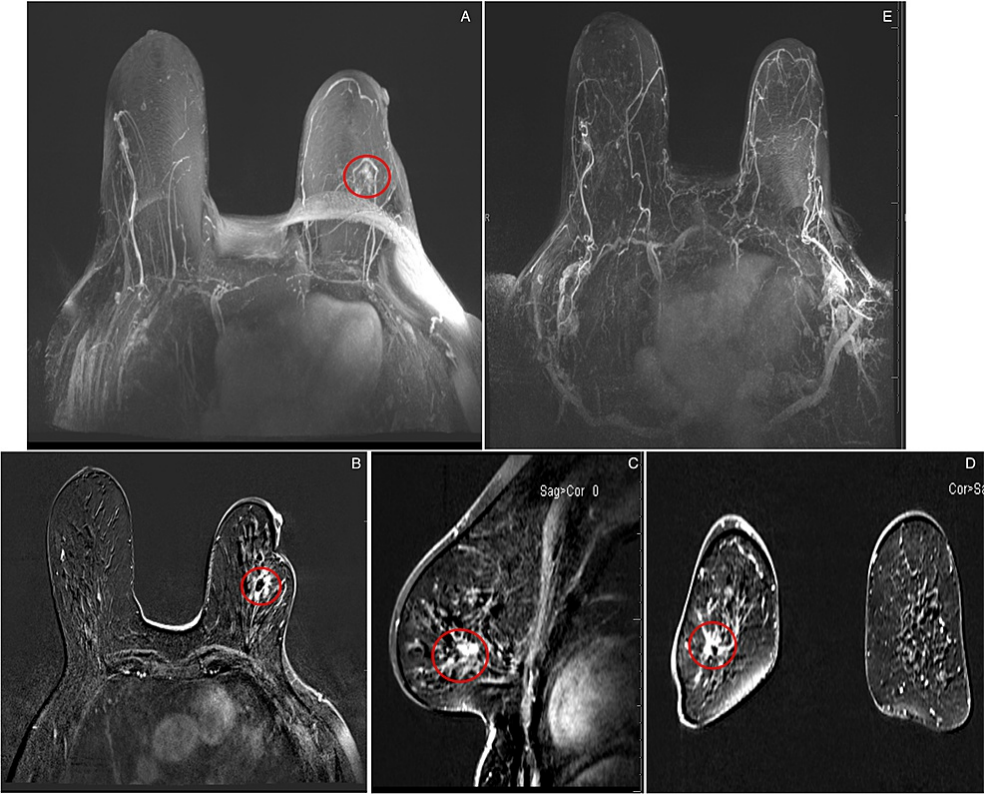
Surveillance MRIs for an asymptomatic 68-year-old patient with a history of breast malignancy who underwent lumpectomy. (A) Axial T1-weighted maximum-intensity-projection contrast-enhanced image shows non-mass enhancement (red circle) at the lumpectomy bed surrounding the fat necrosis. Axial (B), sagittal (C), and coronal (D) T1-weighted maximum-intensity-projection contrast-enhanced with subtraction image shows non-mass enhancement (red circle), measuring 26 × 16 × 22 mm at the 3 o'clock, 4 cm from the nipple surrounding the fat necrosis. (E) Axial T1-weighted maximum-intensity-projection contrast-enhanced MRI performed one year ago shows no abnormal enhancement. MRI: magnetic resonance imaging
